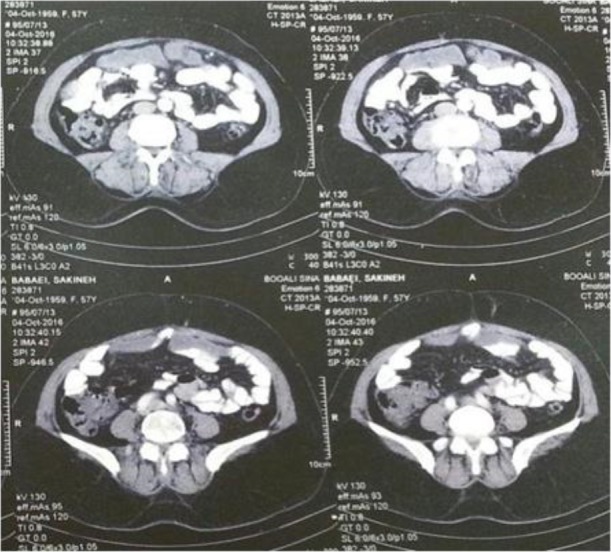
Spiral pelvic CT scan with IV&ORAL contrast shows multiple hypodense cysts in peritoen
